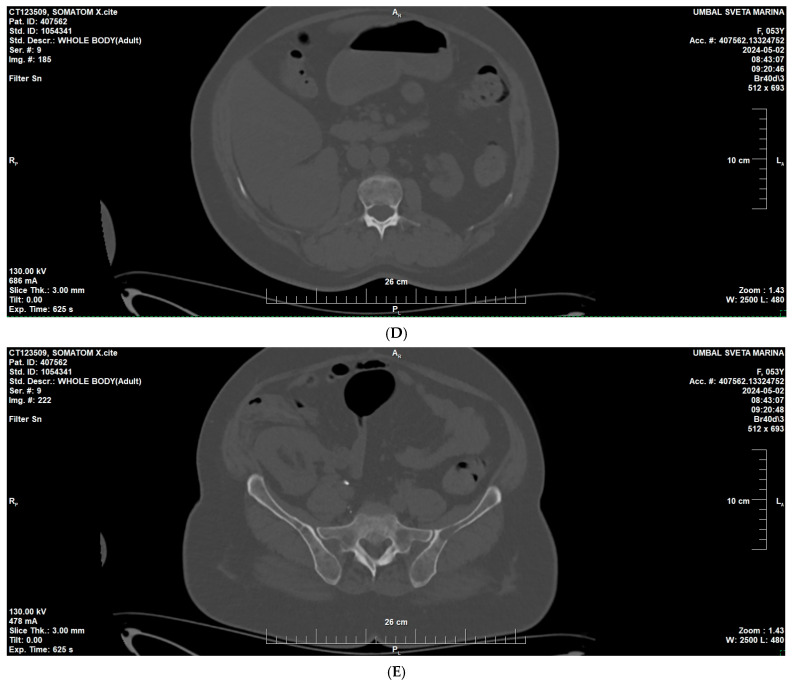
Low-dose whole-body CT findings at diagnosis of multiple myeloma—multiple osteolytic lesions. (A) Skull CT showing multiple “punched-out” osteolytic lesions in the calvaria. (B) CT of the right clavicle demonstrating an expansile osteolytic lesion with cortical thinning. (C) CT of the thorax and lumbal region (D) showing osteolytic lesions involving the vertebral bodies and ribs. (E) CT of the pelvis demonstrating multiple osteolytic foci in the iliac and pubic bones.
